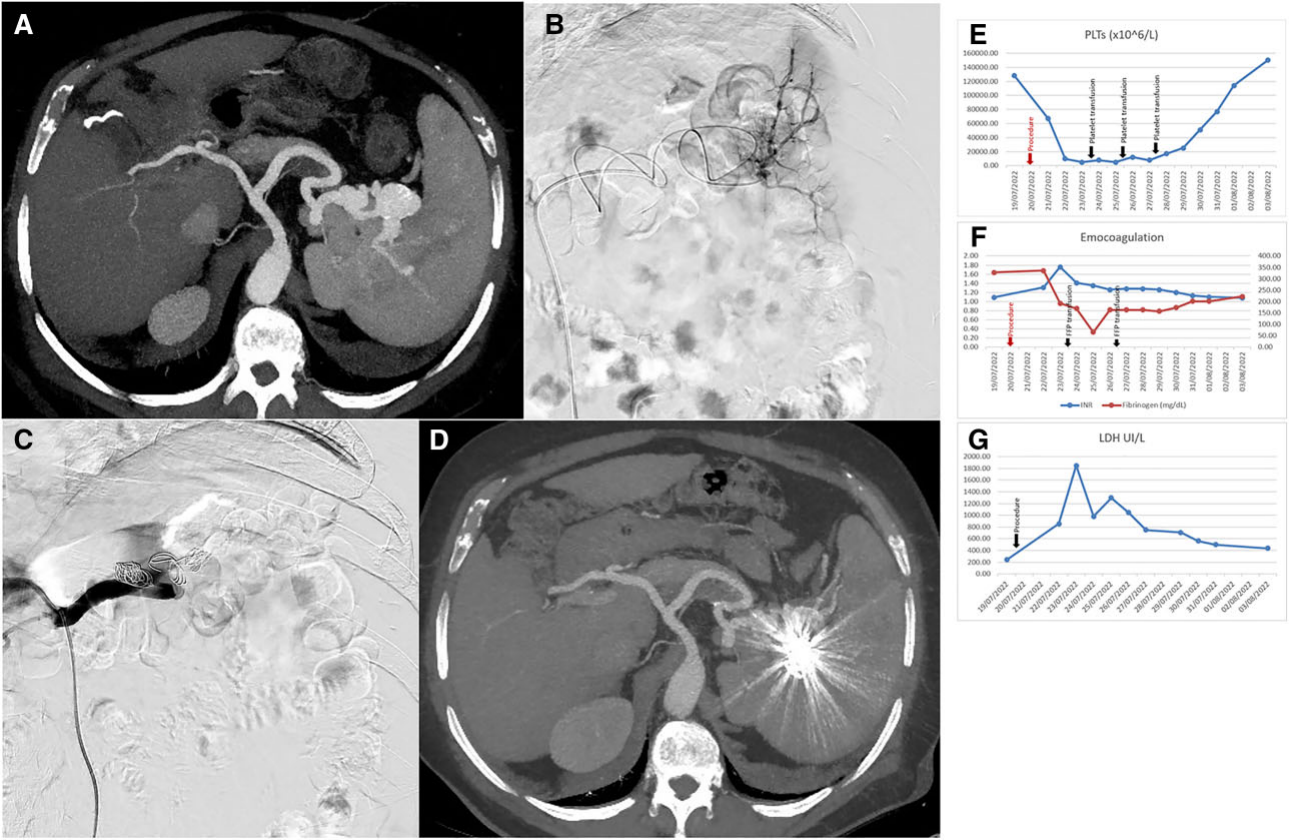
The clinical data of the case. (A) Preoperative computed tomography sub-volume axial maximum intensity projection reconstruction of arterial phase showing two saccular aneurysms of the middle (diameter 16 mm) and distal (diameter 30 mm) portion of the splenic artery. (B) Digital subtraction angiography with catheterization of the hilar portion of the splenic artery by using a coaxial microcatheter. (C) Due to the severe tortuosity of the splenic artery, the “packing” by coils of the distal aneurysm was impossible and therefore the splenic artery was occluded by coils in the middle portion. After releasing three coils and achieving slowing flow, the occlusion of the artery was performed by using a transcatheter injection of 2,500 UI of thrombin. (D) Sub-volume axial maximum intensity projection reconstruction of the arterial phase of computed tomography performed 4 days after the procedure. Coils are migrated in the distal splenic artery. In the portal phase of the exam, no relevant alterations of splenic parenchyma were detected (images not shown). (E) Platelet count (PTL) trend. Black arrows indicate platelet transfusion. (F) Coagulation parameter trend: international normalized ratio (INR) and fibrinogen. Black arrows indicate fresh frozen plasma (FFP) transfusions. (G) Lactate dehydrogenase (LDH) trend.
